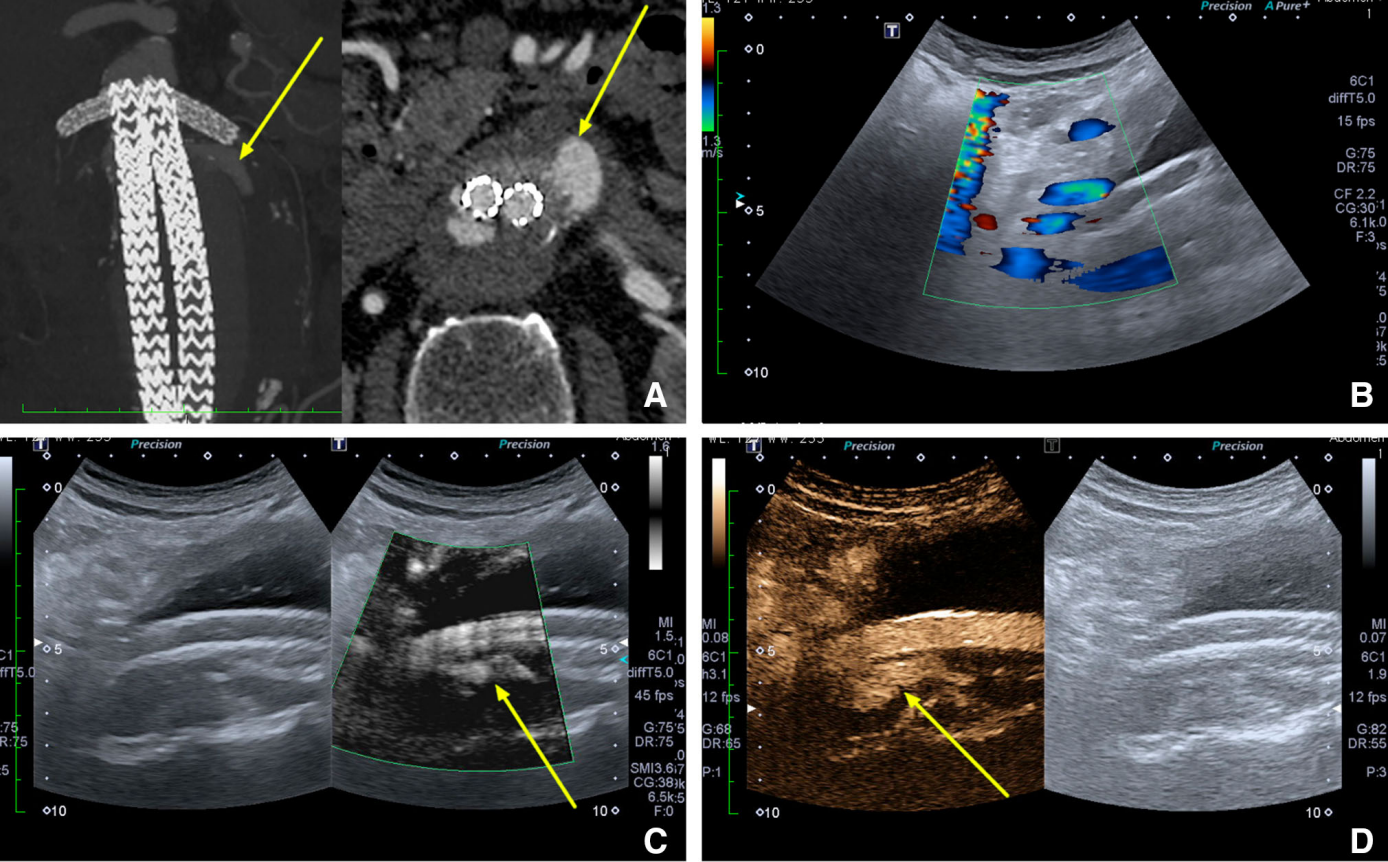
A type I endoleak (yellow arrow) according to four different imaging methods of an abdominal aortic aneurysm: A Computed Tomography Angiography, B Color Doppler Ultrasound, C monochrome Superb Micro-vascular Imaging, D Contrast-Enhanced Ultrasound
